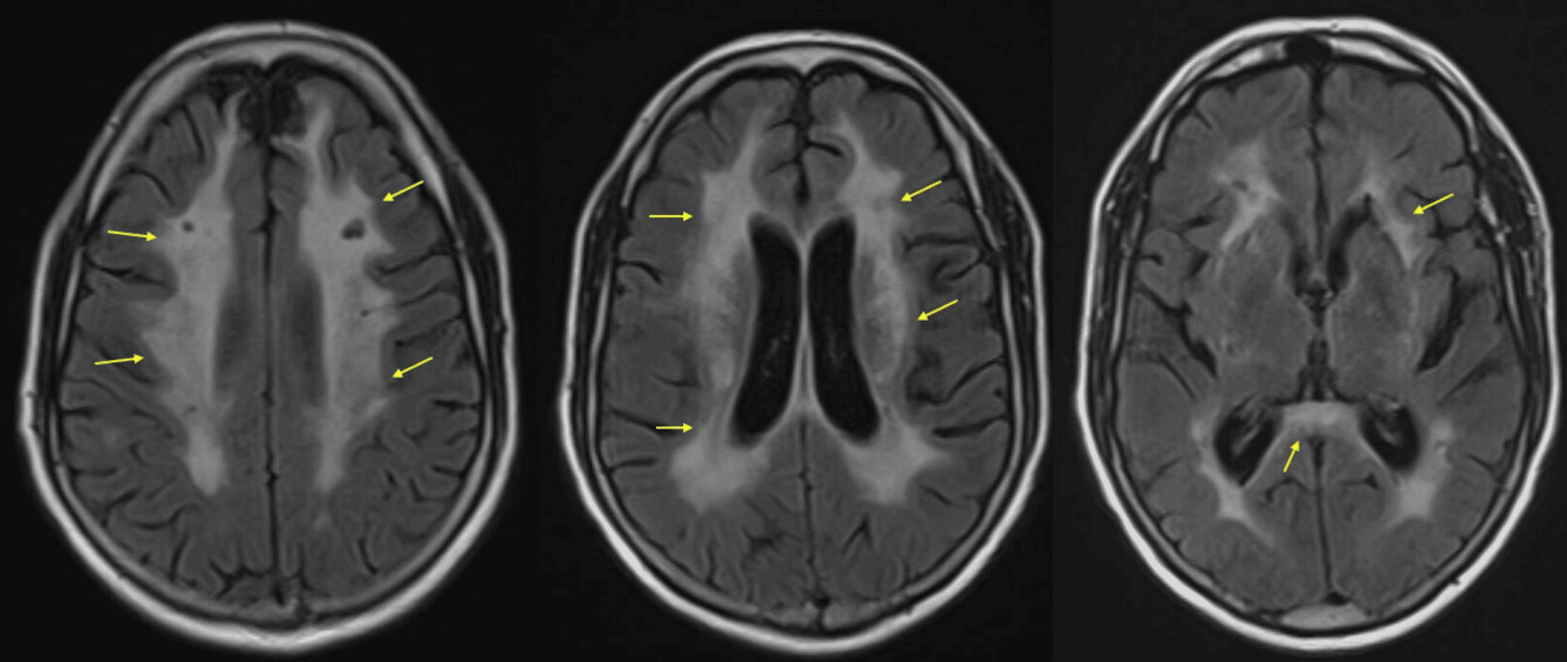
MRI Brain Without Contrast T2 FLAIR Sequence Shown in the image is a series of MRI brain without contrast T2 FLAIR sequence axial images. The panel shows three images with extensive, confluent periventricular white matter T2/FLAIR hyperintensities indicated by the yellow arrows. There are multiple remote lacunar infarcts within the areas of white matter signal abnormalities including bilateral paramedian frontal lobe insults. MRI: magnetic resonance imaging, T2 FLAIR: T2 fluid-attenuated inversion recovery.
